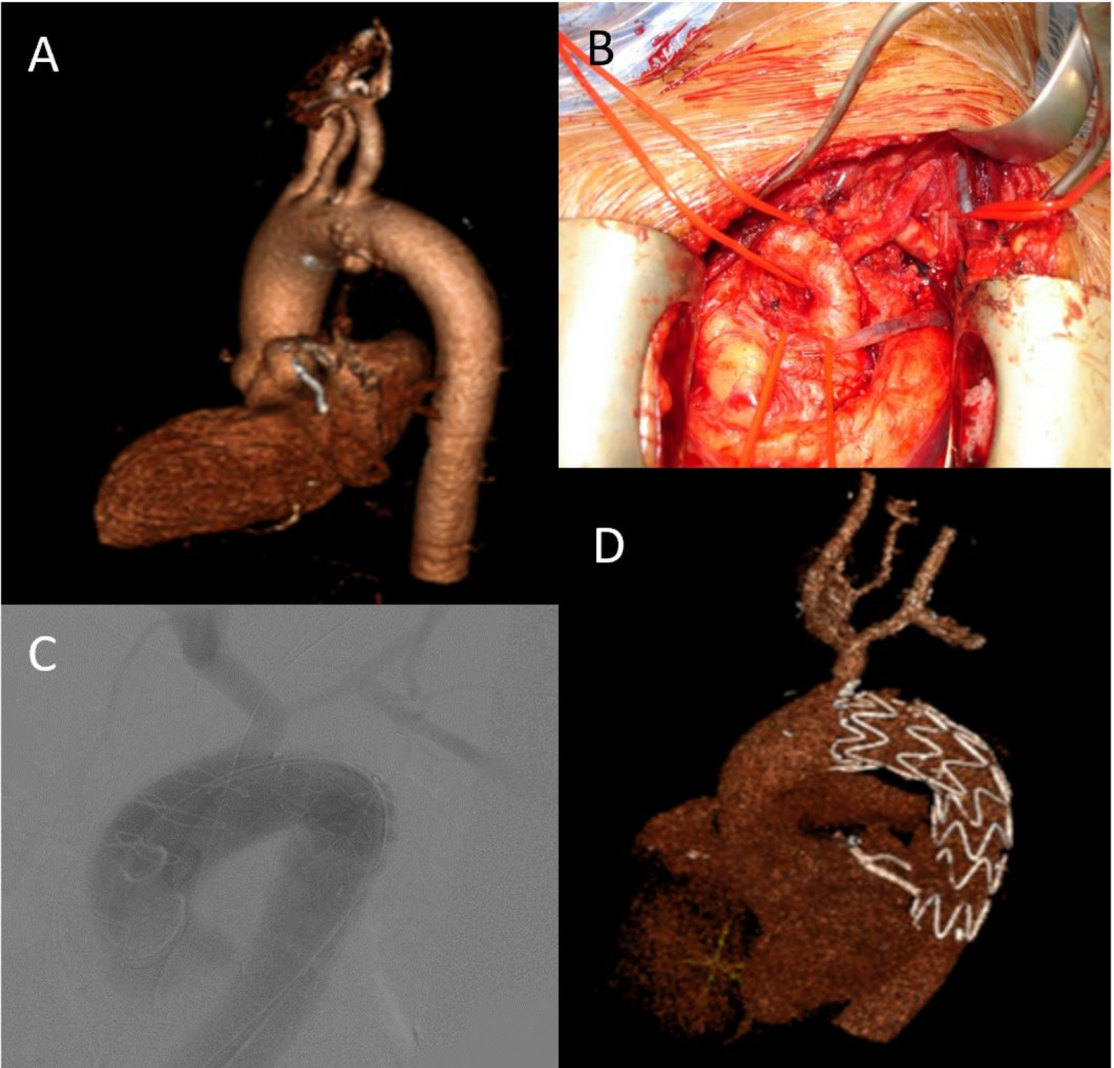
Preoperative, intraoperative and postoperative imaging. A- Preoperative volume-rending 3D computed tomography reconstruction of aorta showing penetrating atherosclerotic ulcer. B- Intraoperative, native double debranching of left subclavian artery and left carotid artery to the brachiocephalic trunk via mini-sternotomy. C- Intraoperative, Digital subtraction angiography (DSA) RelayPro in zone 1. D- Postoperative, volume-rending 3D-CT reconstruction of aorta of RelayPro in zone 1.
